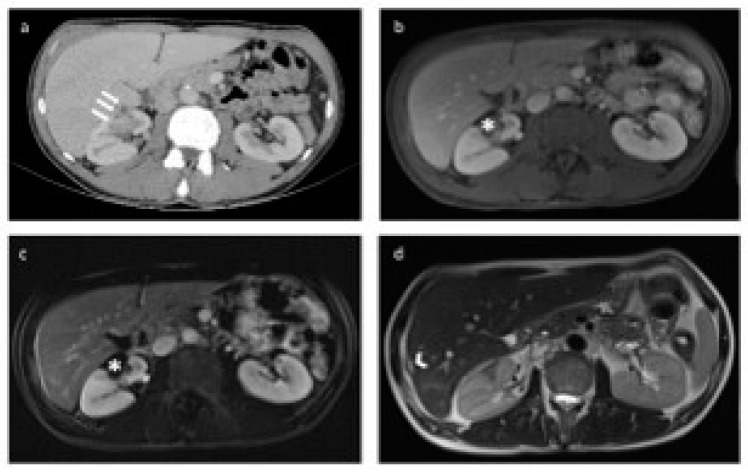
Preoperative computed tomography (CT) and postoperative magnetic resonance (MR) images of the patient treated with percutaneous microwave ablation therapy (MWAT) via transhepatic approach. (a) In the axial-plane postcontrast portal venous phase CT image, a lesion (white arrow) is observed in the anteromedial aspect of the middle pole of the right kidney before the operation, in close proximity to the renal vascular structures. (b,c) No residual lesion was detected in the tumor bed (asterisk) after percutaneous MWAT on axial postcontrast portal venous phase and subtracted T1-weighted MR images. (d) In the axial-plane T2-weighted MR image, the loculation is observed in the neighborhood of the liver segment 7-6 junction, which is compatible with the transhepatic ablation trace (arrowhead).
